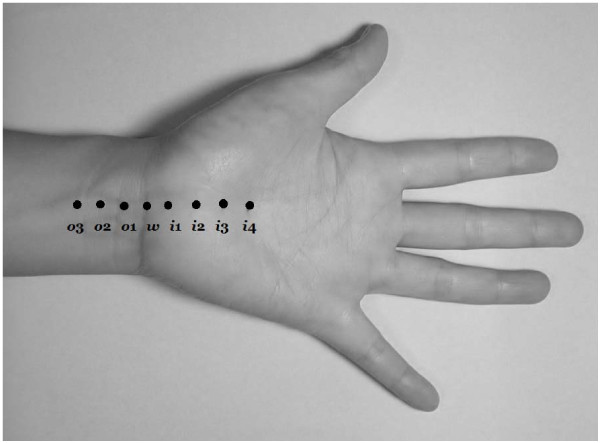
The 8 points (*i*4, *i*3, *i*2, *i*1, *w*, *o*1, *o*2, and *o*3) for recording in both the “inching test” and ultrasonography.*i*4, *i*3, *i*2, *i*1 represent levels at 4, 3, 2, and 1 cm distal to the wrist crease in the inlet of the carpal tunnel; *w* represents the level of the wrist crease and *o*1, *o*2, and *o*3 represent levels at 1, 2, and 3 cm proximal to the wrist crease in the outlet of the carpal tunnel.
